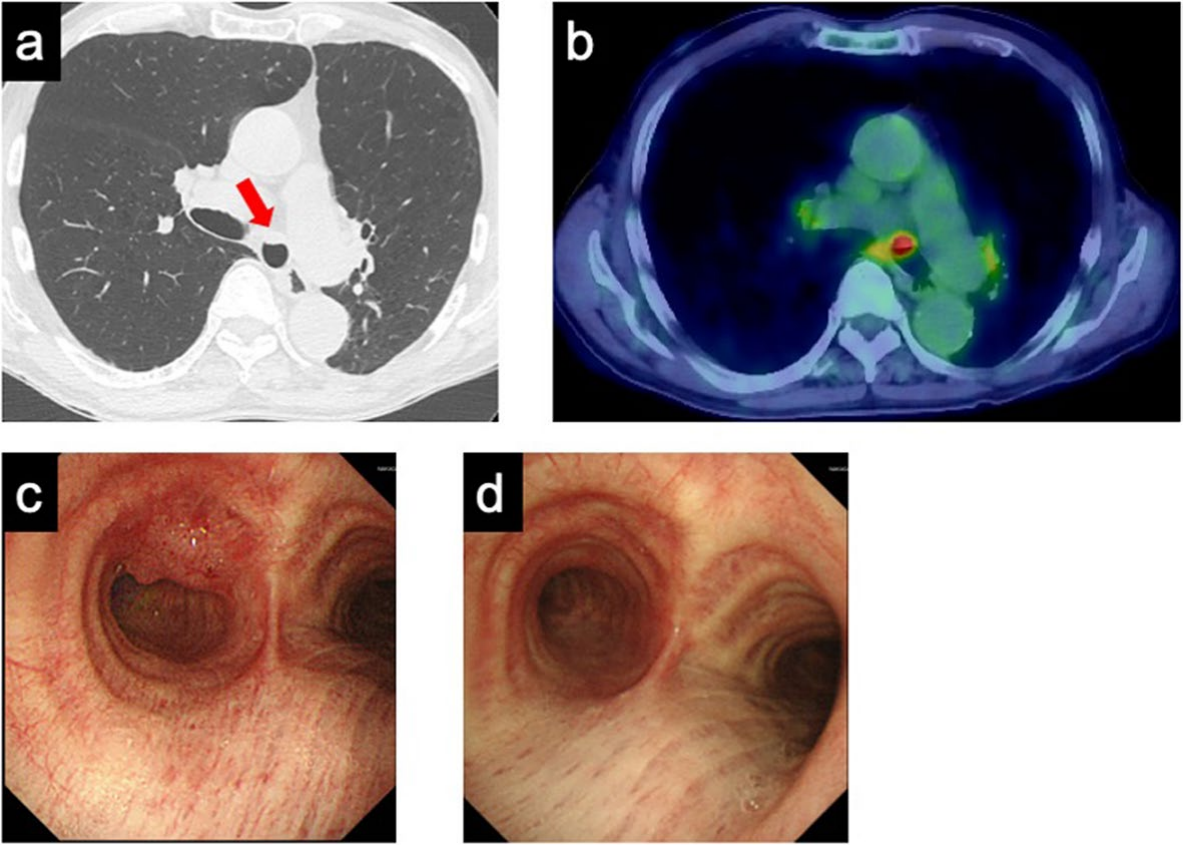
a CT findings showed the endobronchial metastasis at the left main bronchus (arrow). b PET-CT revealed an uptake in the lesion [SUV_max_ 7.4]. Bronchoscopic image of c before irradiation and d after irradiation
